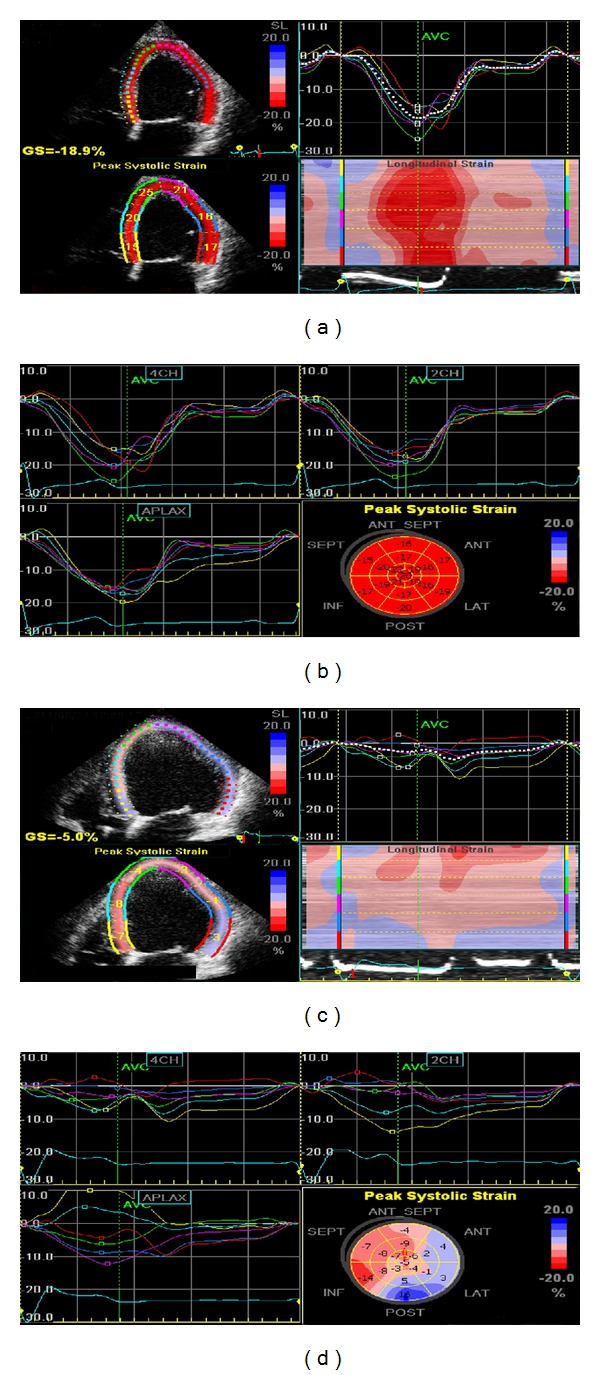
Examples of strain imaging using speckle tracking echocardiography. On the top row are images from a normal healthy volunteer (apical 4-chamber view on the left and summary strain scores with bulls-eye plot on the right), and on the bottom row are corresponding images from a patient with severe ischaemic cardiomyopathy. Note that the healthy volunteer has normal longitudinal strain in all segments with a global strain score of −18.9%, whereas the cardiomyopathy patient has highly abnormal strains with a significantly depressed global strain score of just −5.0%.
